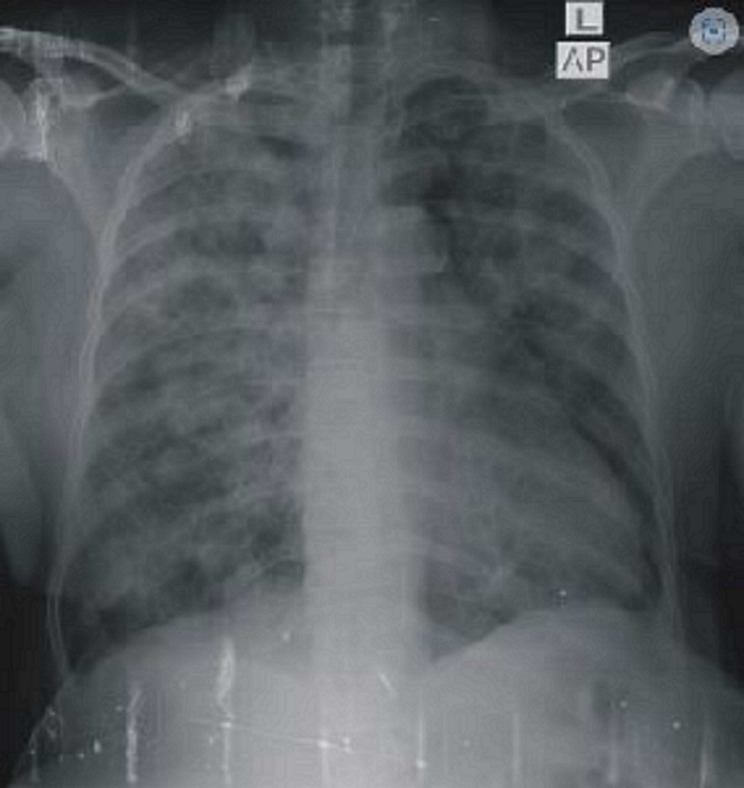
plain chest X-ray (anterior-posterior view) showing features of bilateral pulmonary edema
